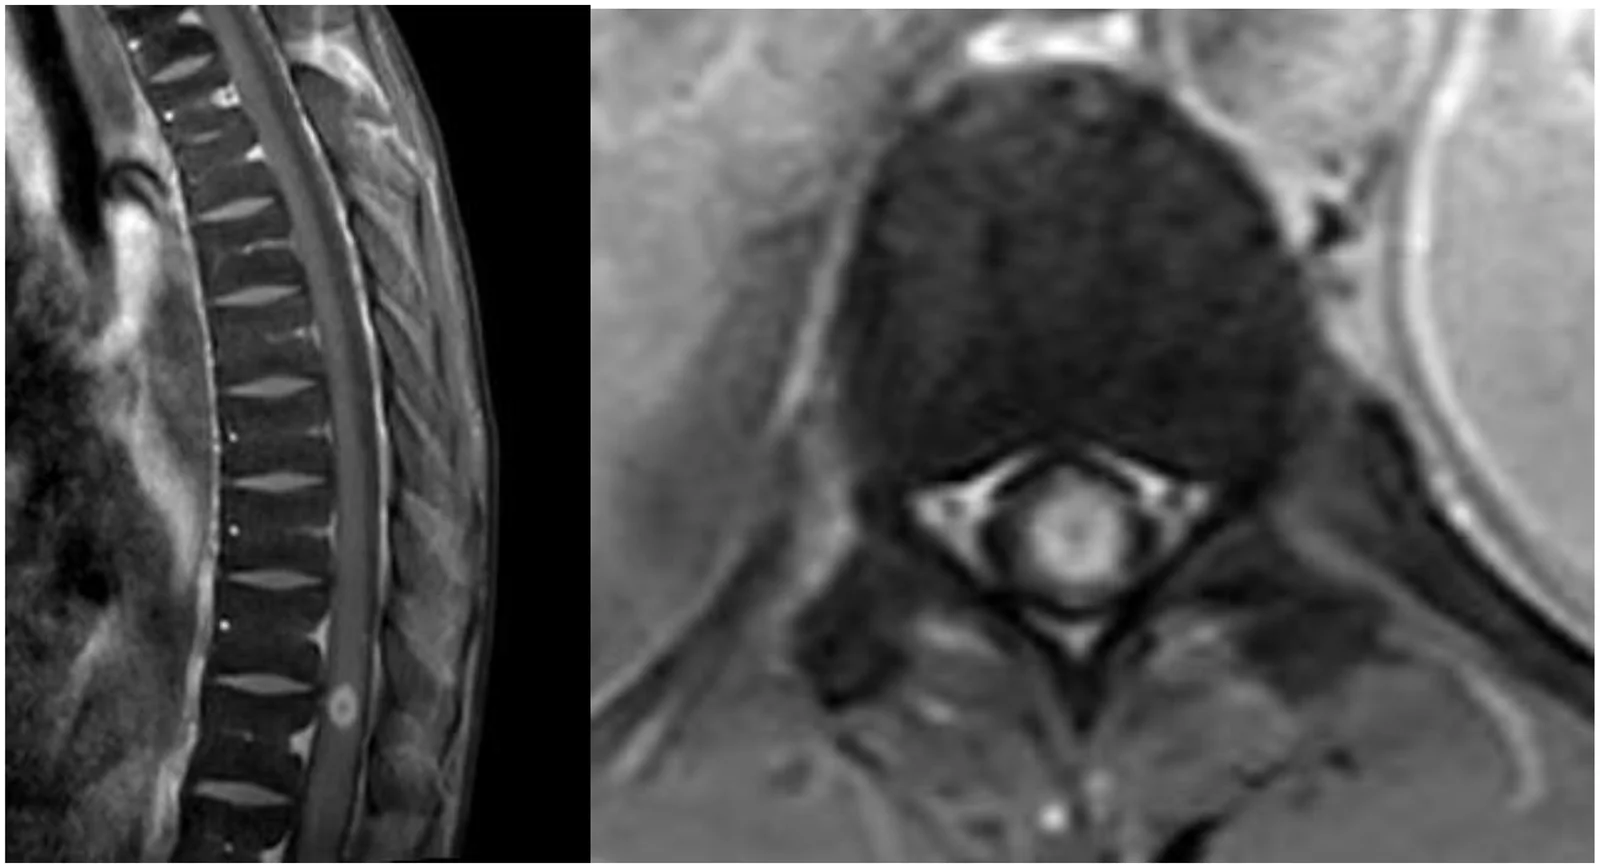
Sagittal and axial postcontrast T1-weighted MRI demonstrate a centrally located lesion with complete peripheral (ring-like) annular enhancement following administration of paramagnetic contrast, consistent with a rim-enhancing pattern. The enhancement delineates the lesion margins with a nonenhancing central component, suggesting central necrosis.
